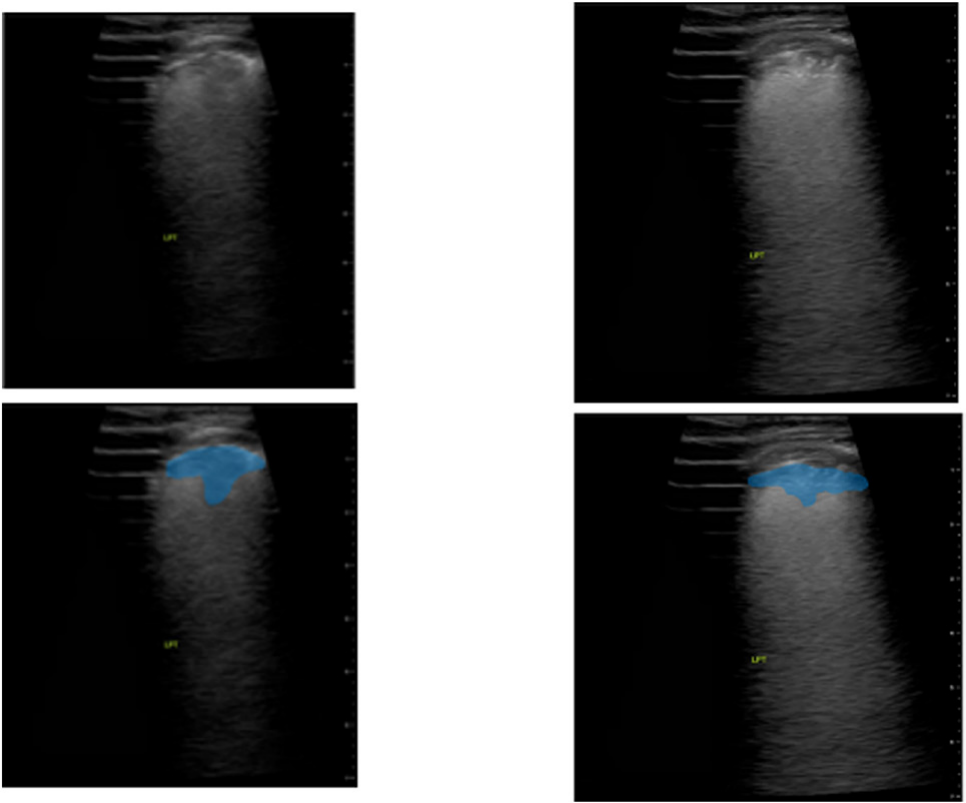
Figure 3. Lung POCUS (Point of Care Ultrasound) findings of bilateral Consolidation and non-consolidation pattern and bilateral interstitial pattern (only finding on CXR)
